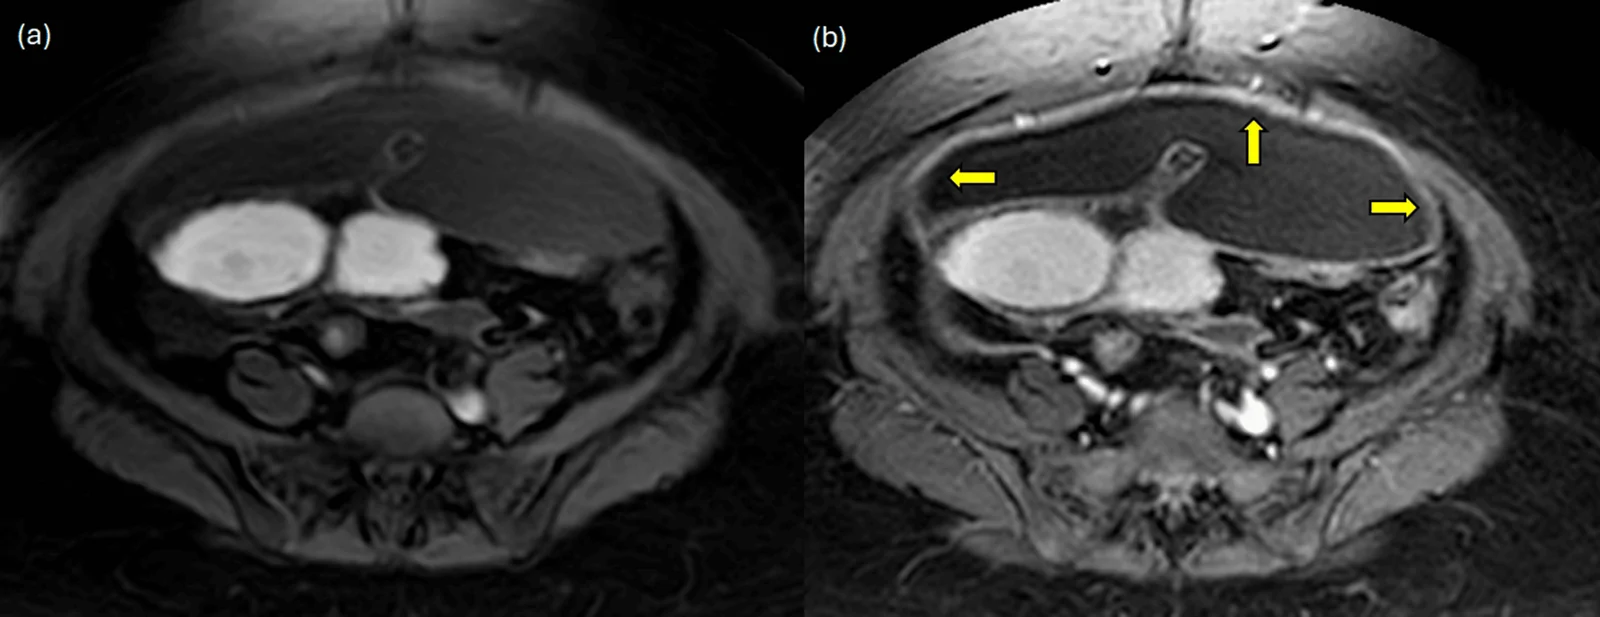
Pelvic MRI: (a) fat-suppressed T1-weighted and (b) contrast-enhanced T1-weighted sequences The lesion is intrinsically hyperintense on the pre-contrast T1 fat-saturated sequence due to its methemoglobin content, without enhancement of any internal components on the contrast-enhanced sequence. Note the enhancement of the parietal peritoneum due to chronic inflammation and irritation from blood products (yellow arrows). MRI: magnetic resonance imaging
